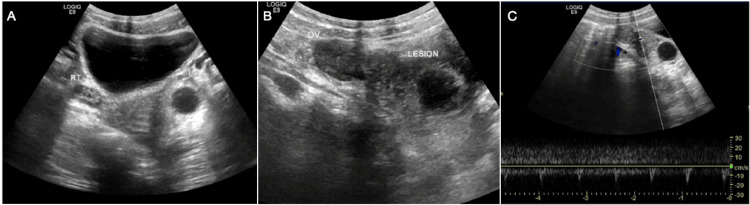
Ultrasound images of isolated fallopian tube torsion Image A shows a normal right ovary appearance and a cyst in the left adnexa. Image B shows the left adnexa, where the left ovary is noted to be pushed anteriorly with no obvious sonographic signs of torsion; posterior to it, there is a hypoechoic lesion containing an anechoic cyst. Image C shows vascularity within the lesion.
